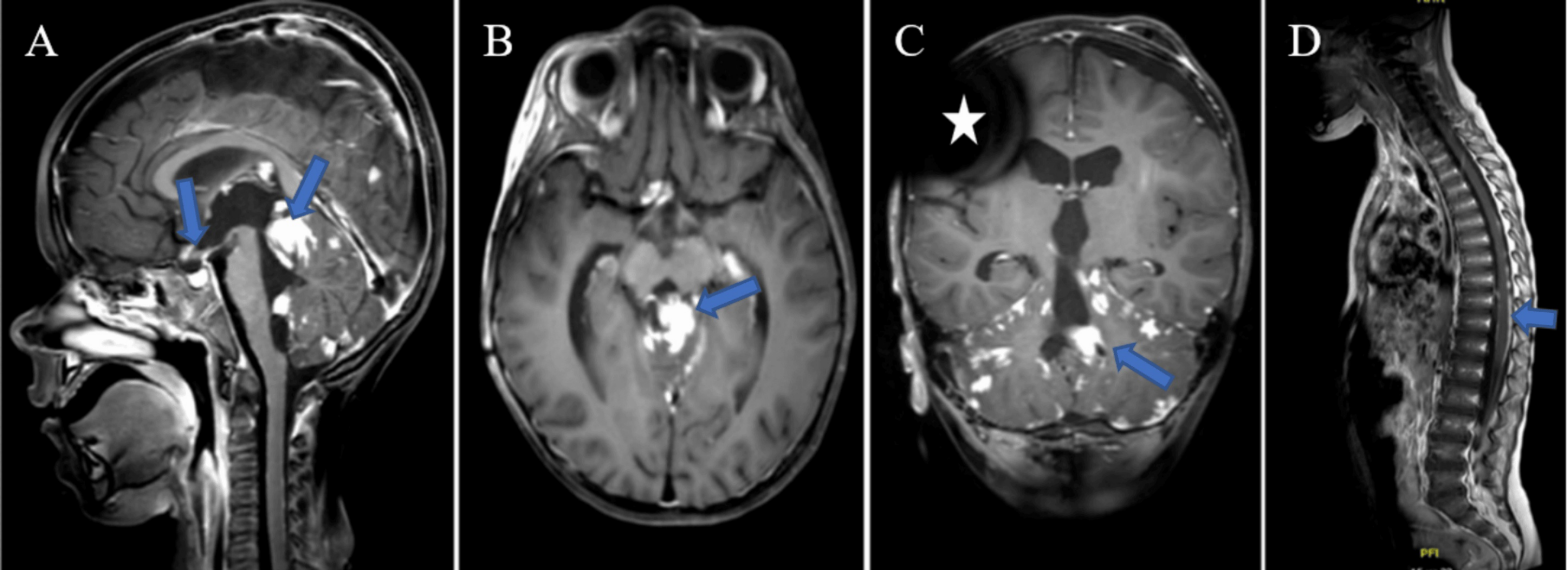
T1 MRI of the CNS three months after the start of IPCT Shows significant tumor shrinkage compared to the first MRI study. In the coronal section (C), a hypointense artifact is seen resulting from the VPS valve (white asterisk). CNS, central nervous system; IPCT, intensive polychemotherapy; VPS, ventriculoperitoneal shunt
